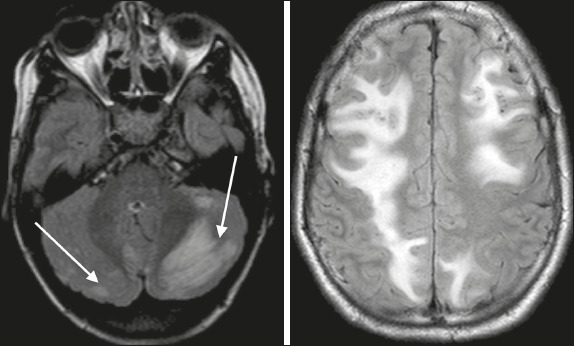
Brain MRI. Axial T2-weighted FLAIR sequence showing lesions in atypical regions:
